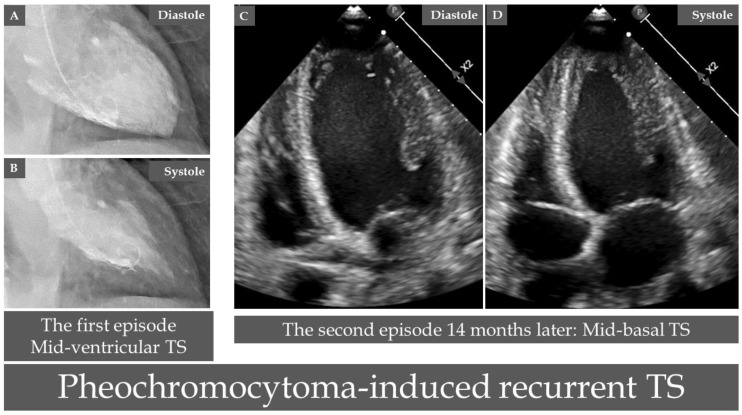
A case of recurrent takosubo syndrome (TS) induced by a pheochromocytoma. Contrast left ventriculography reveals mid-ventricular pattern of TS during the first episode ((A), diastole and (B), systole). During recurrent TS 14 months after the first episode, echocardiography reveals typical mid-basal (inverted) pattern of TS ((C), diastole, and (D), systole). Interestingly, the case was deemed as “type 2 myocardial infarction” during the first episode when pheochromocytoma was not diagnosed.
